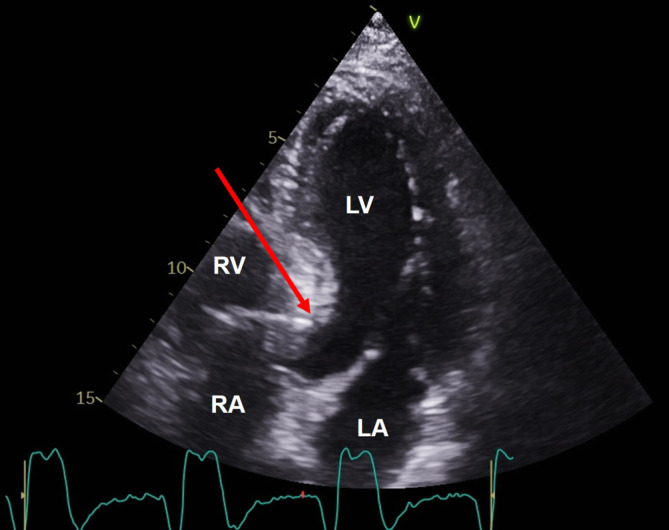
Echocardiography in left bundle branch (LBB) pacing. In the apical view, the tip of the LBB pacing lead (*arrow*) is visible just under the left ventricular endocardial surface. *LA* left atrium, *LV* left ventricle, *RA* right atrium, *RV* right ventricle
